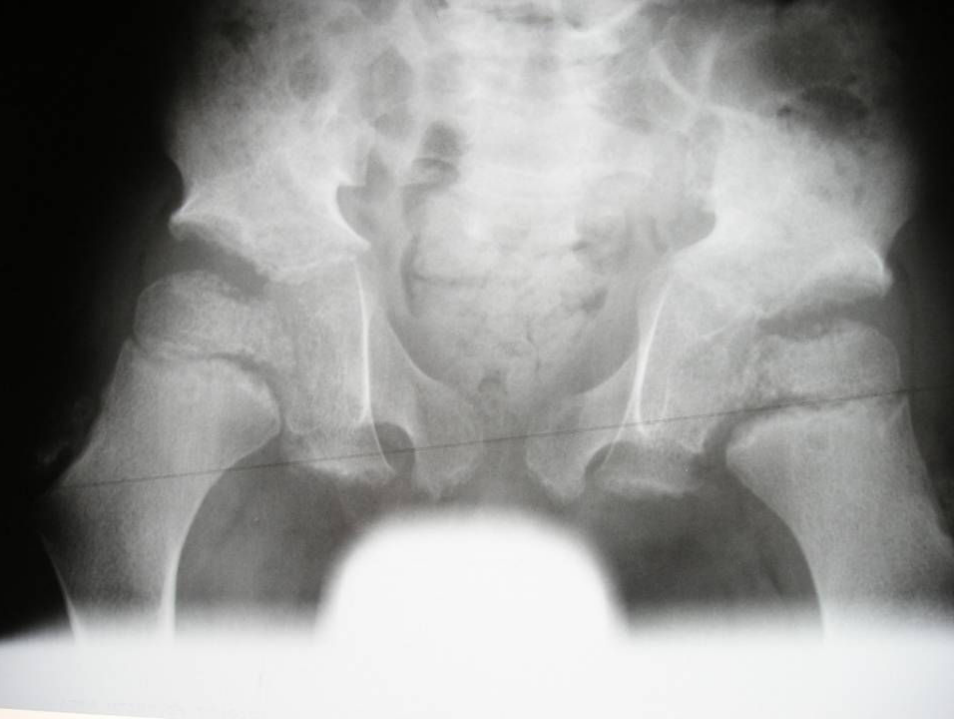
Anteroposterior pelvis radiograph showed, coxa valga, a hypoplastic ileae, acetabulo-femoral and metaphyseal dysplasia, with the propensity for the development of slipped epiphyses associated with multiple epiphyseal fragmentations/stippling. The latter might lead for the development of Perthes disease. Early signs of early osteoarthritis were evident.
